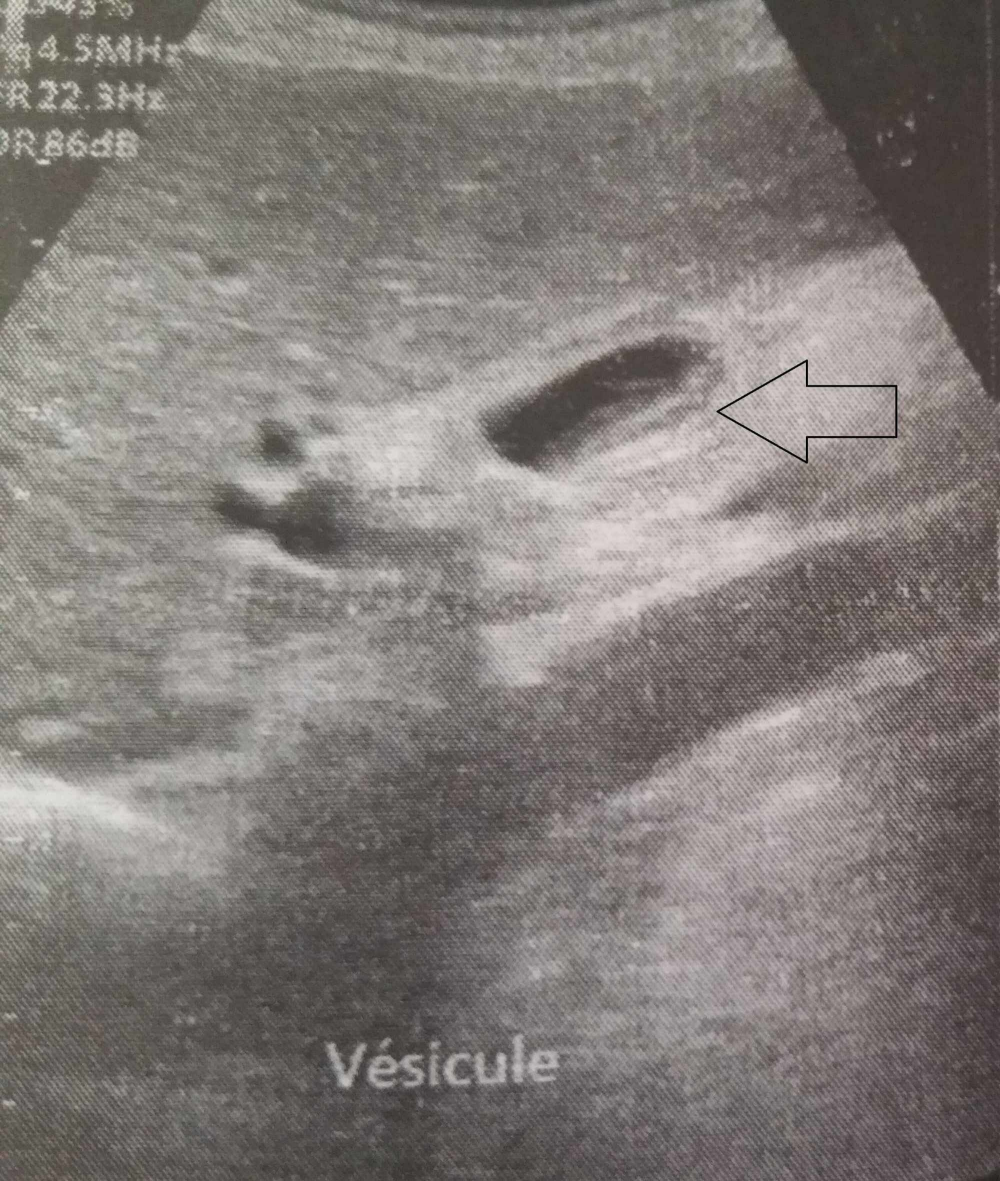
Ultrasonography showing thickened gallbladder wall (black arrow).
